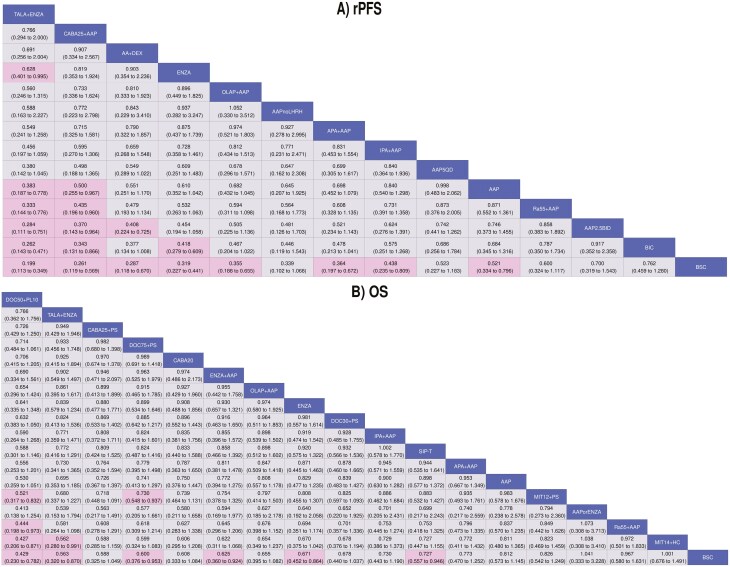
Random-effects league tables for rPFS and OS. Abbreviations: OS, overall survival; rPFS, radiographic progression-free survival. Values are HRs (95% credible interval) for relative effectiveness for all possible pairs of treatments in the network. HR < 1 implies that column is better than row. Pink squares are statistically significant. For full list of treatment regimens, refer to Appendix B.
